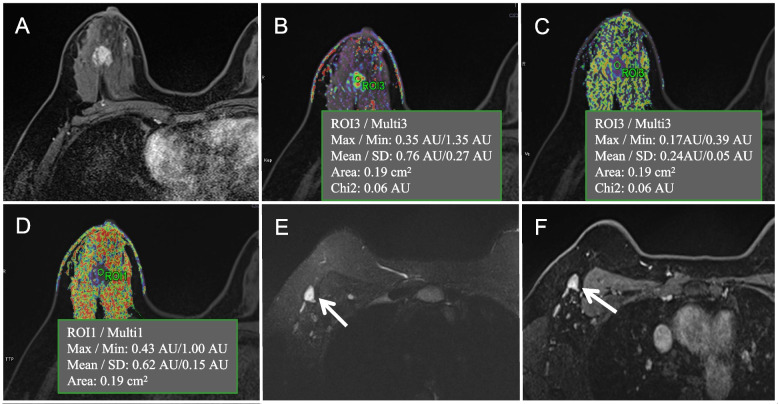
One example of the point-based scoring system for predicting breast cancer patients without axillary lymph node metastasis. A ~ F are MRI images of a female right breast cancer patient with invasive ductal carcinoma who was positive for human epidermal growth factor receptor-2, negative for estrogen receptor, AJCC stage III, and T2 stage. An axial contrast-enhanced T_1_WI image (A) shows a tumor with a maximum diameter of 2.3 cm located in the lower-outer quadrant. The mean values of K_ep_, V_e,_ and TTP from the corresponding pseudocolor images of K_ep_ (B), V_e,_ (C), and TTP (D) were 0.760 min^−1^, 0.190, and 0.620 min, respectively. Breast MRI was suspicious for axillary lymph node metastasis based on the following features: axial T_2_WI with fat suppression imaging (E) and axial contrast-enhanced T_1_WI imaging (F) showing cortical thickening, an oval shape and a long-to-short axis ratio of 1.4 for a maximal lymph node (white arrow) in axillary level I (nodes lateral and inferior to pectoralis minor muscle). However, the risk of axillary lymph node metastasis assessed by the scoring system was 17.0, with an axillary lymph node metastasis probability of 26.9%. The final postoperative pathology report showed that this tumor had no axillary lymph node metastasis
